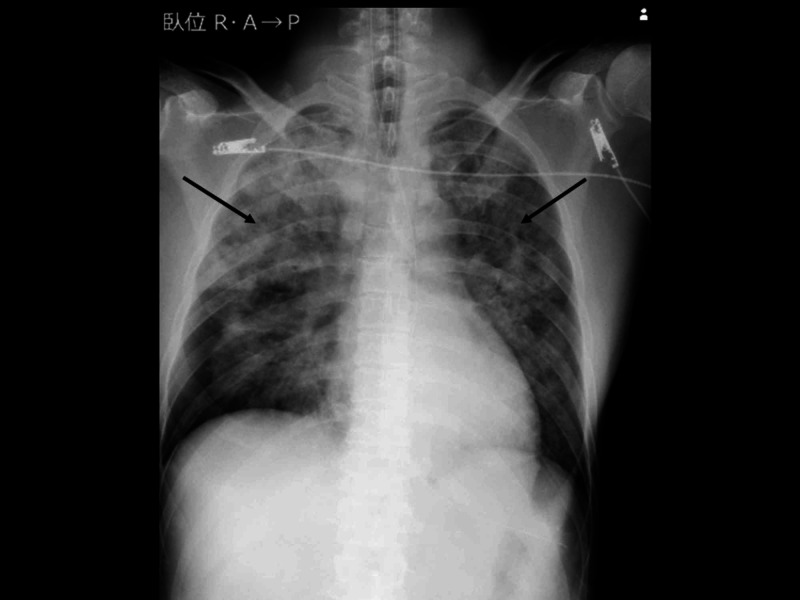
Chest X-ray on arrival The image shows a bilateral ground-glass appearance (arrow)
